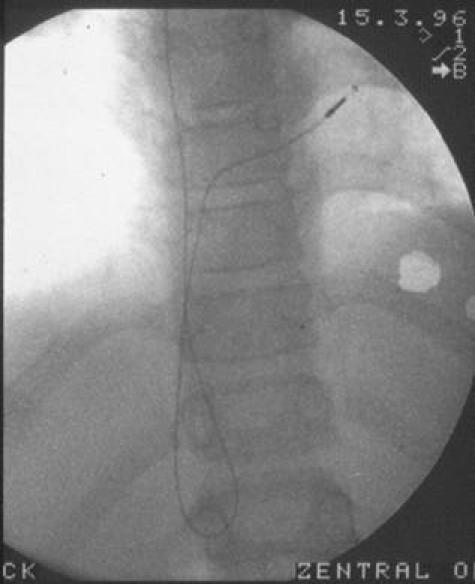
Posteroanterior radiography immediately before reintervention showing the inferior vena caval loop of the pacemaker lead strongly attached to the endothelium. The tip of the electrode is still attached the right ventricular wall. At that time an exit block was predominantly existent.
